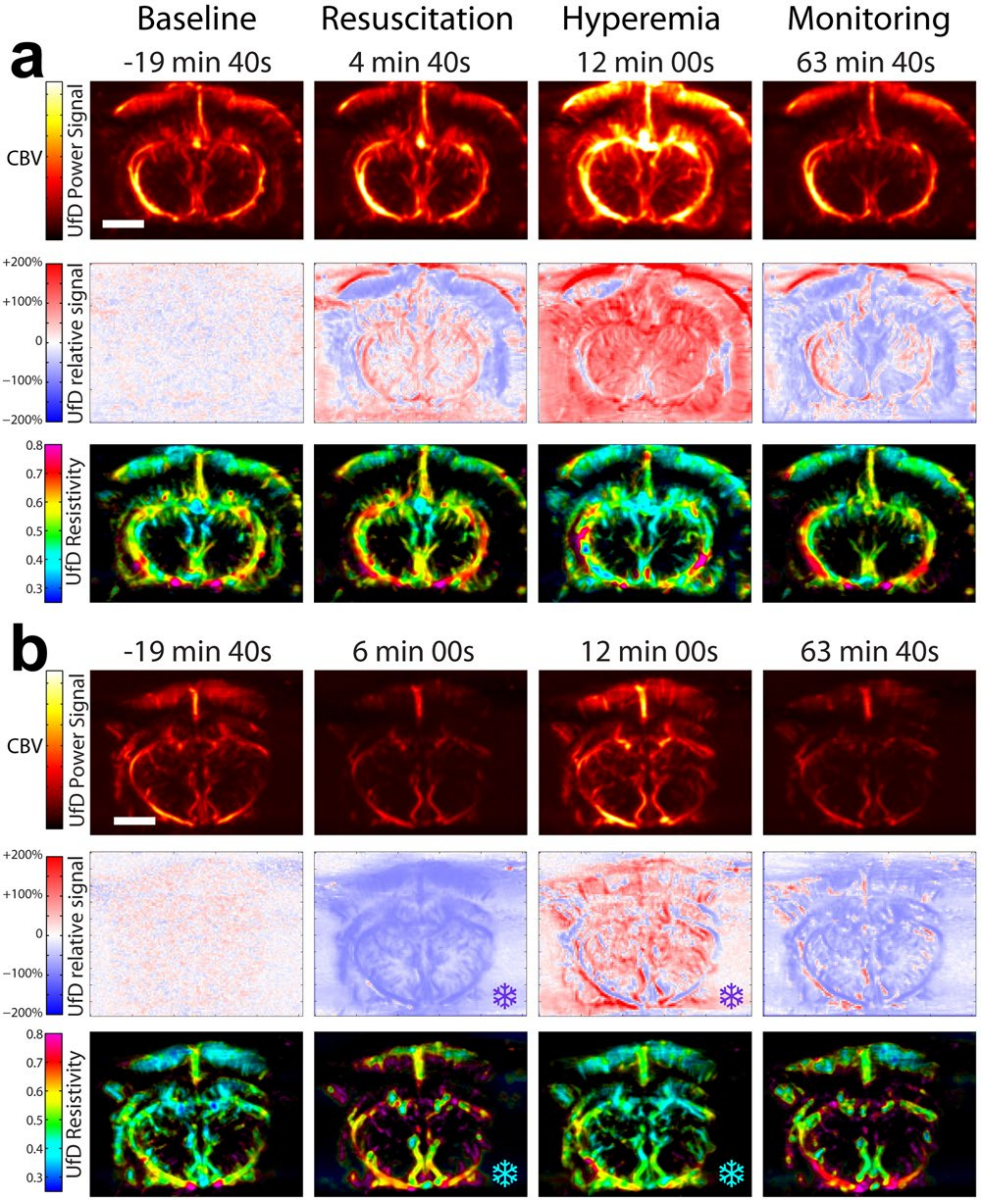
Multiparametric imaging of the brain derived from ultrafast Doppler acquisitions. UfD Power signals (1^st^ row) display intensities proportional to cerebral blood volume. UfD relative signals (2^nd^ row) provide the percentage of increase or decrease in cerebral blood volume with respect to baseline level. UfD resitivity maps (3^rd^ row) the ratio of the range of arterial velocity during the cardiac cycle to the arterial systolic peak velocity. The first column (t = −19 min 40 s) presents baseline state, the second column (t = ~4–6 min) presents the brain status immediately after resuscitation, the third column (t = 12 min) reports brain condition at maximal hyperemia, and the last column (t = 63 min 40 s) presents brain condition one hour after CPR. (a) Control group. (b) TLV group.
